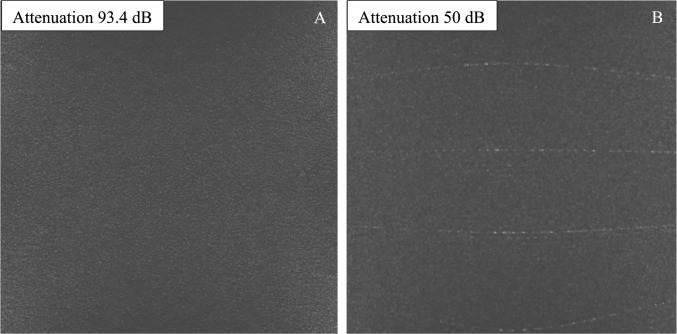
RF interference test images windowed to show ambient noise levels, showing an absence (A) and presence (B) of the characteristic zipper artefact indicating external RF interference. The curved nature of the interference lines towards the edge of the image arise from the MRI system’s geometrical distortion correction. The RF attenuation value at the MRI exam room door for each of these FCs was 93.4 dB and 50 dB respectively. Both FCs were 14 years old with example B displaying RF interference without any neighbouring scanner in operation.
